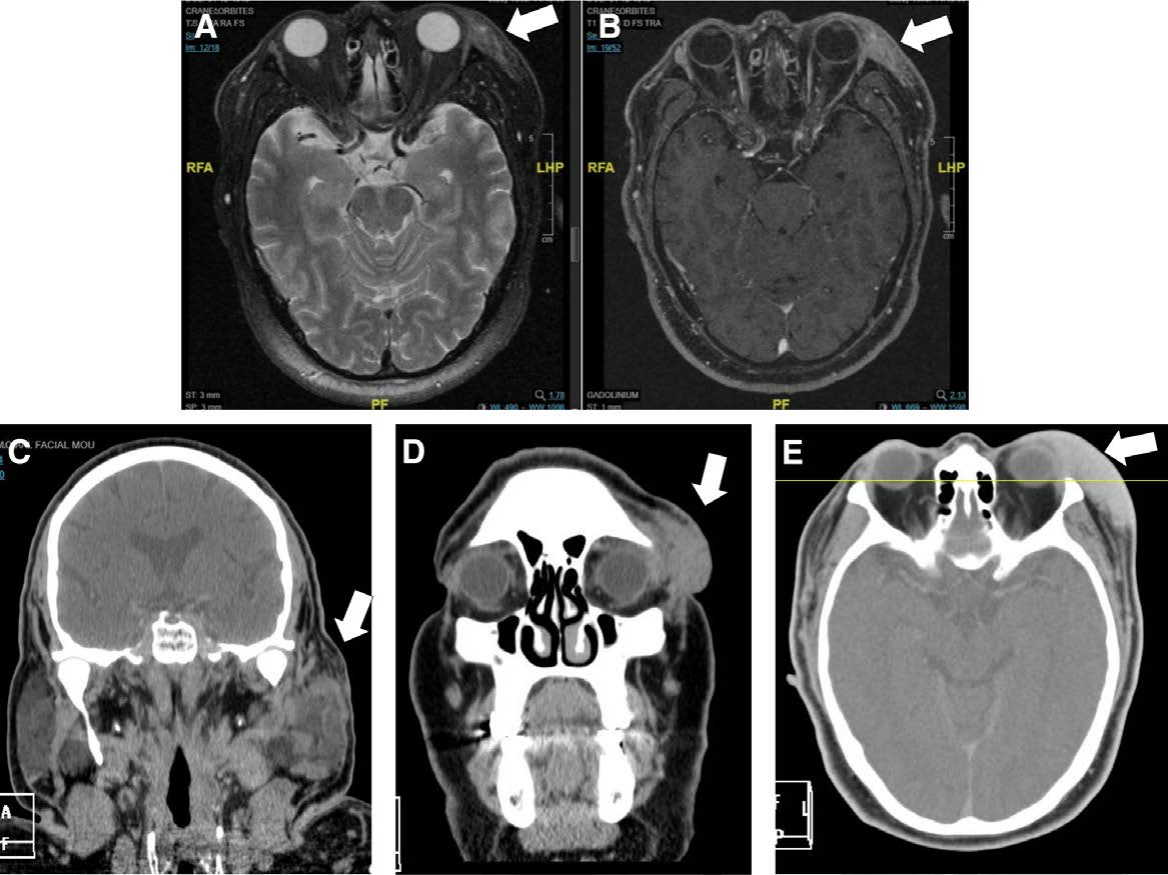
Radiological aspect of KD lesions on MRI and CT scan. Axial MRI: swelling in the upper left eyelid level, appearing as discrete T2 hypersignal (A) and enhanced after injection of gadolinium (B). Deep invasion or bone lysis was not observed. Coronal (C, D) and axial (E) CT scans. Hypertrophy of both parotid glands and within the left one, presence of an infiltrating tissue lesion, and partitioning in places (C). Lesion of the left latero-orbital soft tissue, coming into contact with the eyeball (D), was enhanced after injection (E). CT = computed tomography, KD = Kimura's disease, MRI = magnetic resonance imaging.
